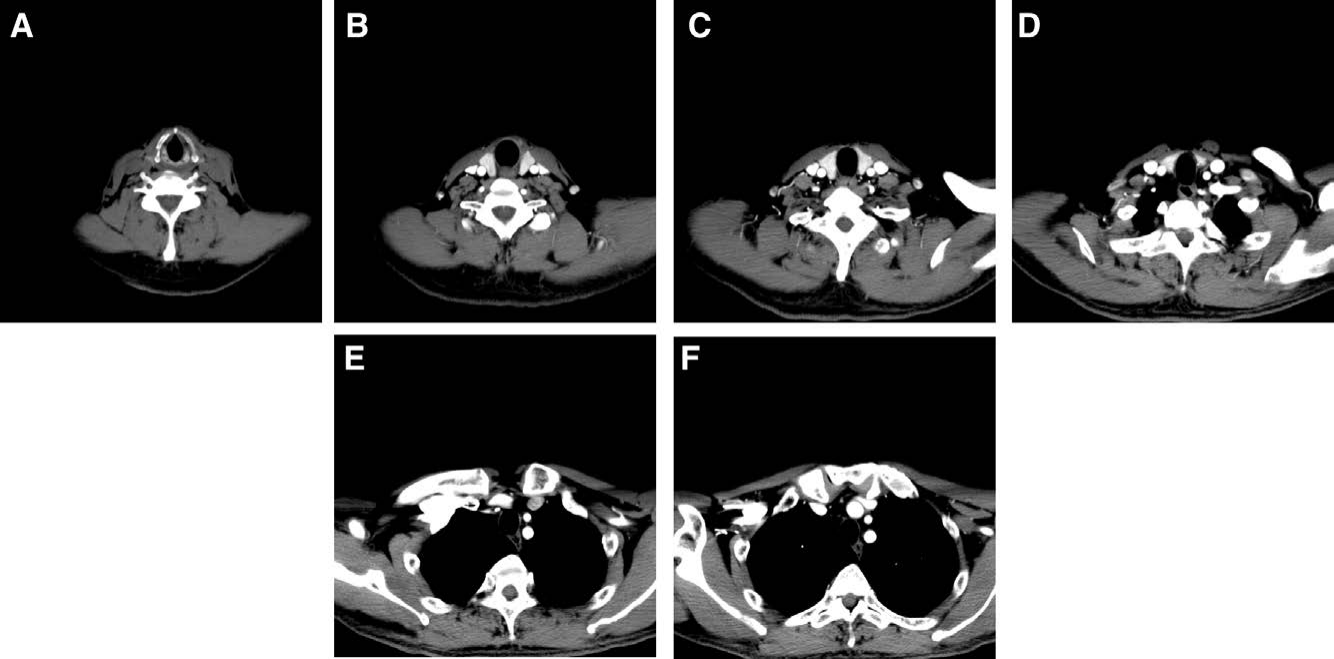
Preoperative neck and chest CT did not reveal a tracheal diverticulum (Fig. 1 A–F). CT = computed tomography.
